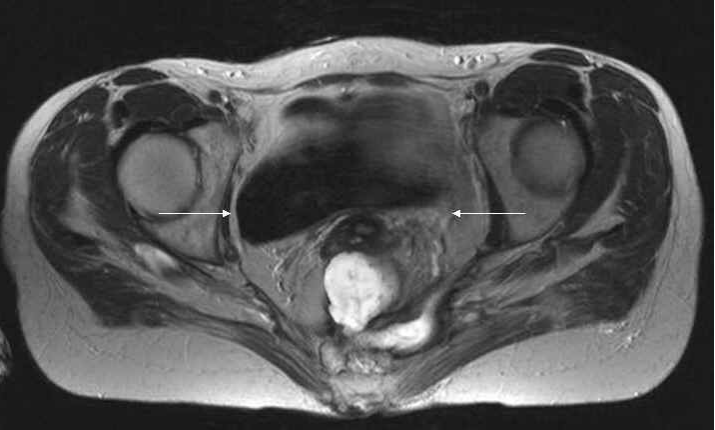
A T2-weighted axial MR image of the pelvis showing an irregular presacral fluid collection (arrows) extending from the cervix to the coccyx and to the sciatic foramen on the left side.
